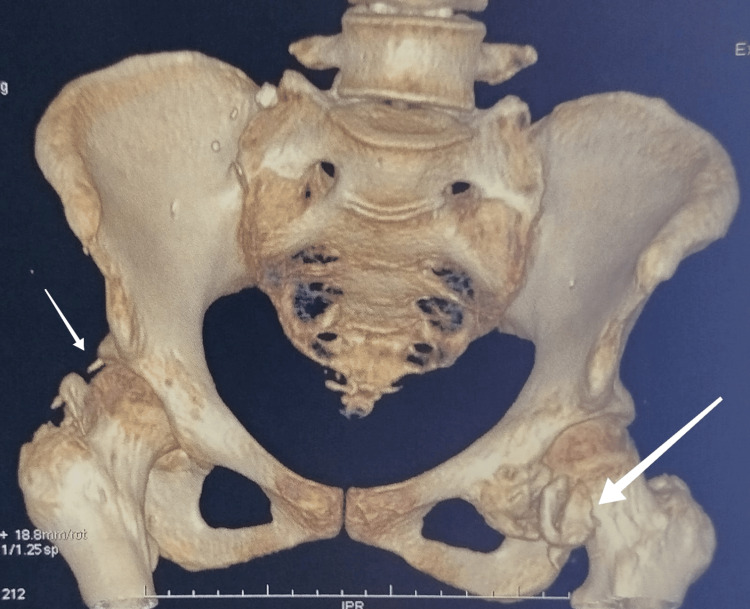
Three-dimensional (3D) reconstructed CT image of the pelvis This reconstruction provides an overview of the extensive bilateral HOs (indicated by white arrows) around both femoral necks and trochanteric regions, consistent with advanced new bone formation. HOs: Heterotopic ossifications
